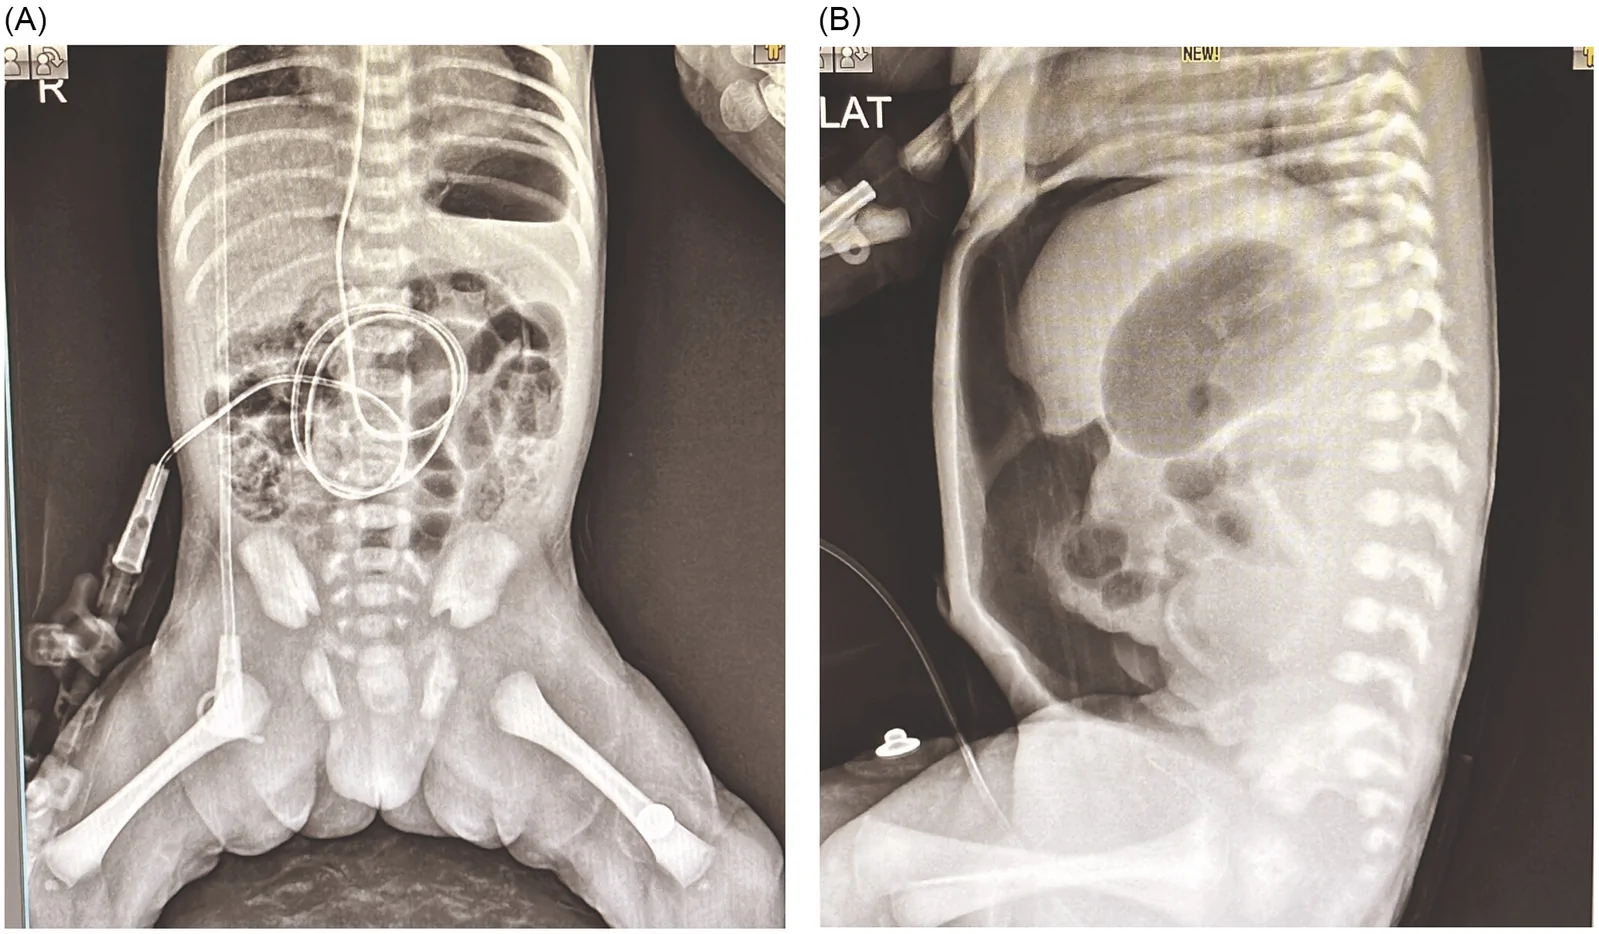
Abdominal radiographs demonstrating the radiological progression of necrotizing enterocolitis (NEC). (
